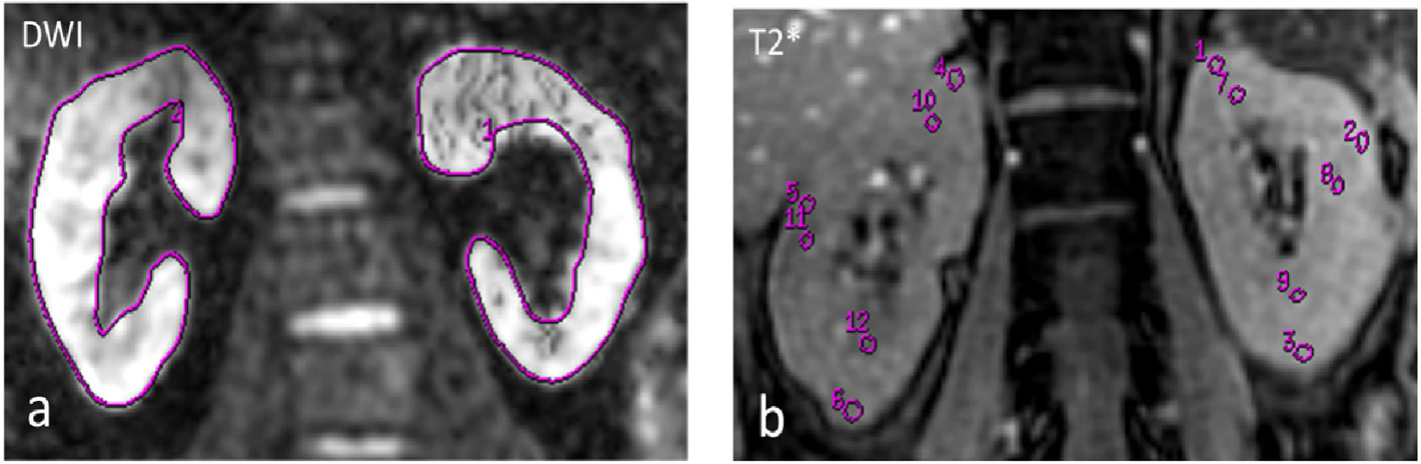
Renal functional MR imaging of Patient B with LN-IV(G)A/C + V. (a) DWI imaging. (b) T2* imaging. The proteinuria was 6.1 g/24 h and serum creatinine was 422 μmol/L, the renal pathology AI was 19 and CI was 4. The differentiation between cortex and medulla became less apparent in T2* imaging. The ADC value of left kidney was 2.14 x10^−3^ mm^2^/s and that of right kidney was 2.20 x10^−3^ mm^2^/s, the R2* values of cortex and medulla were 10.79/s and 8.67/s respectively.
